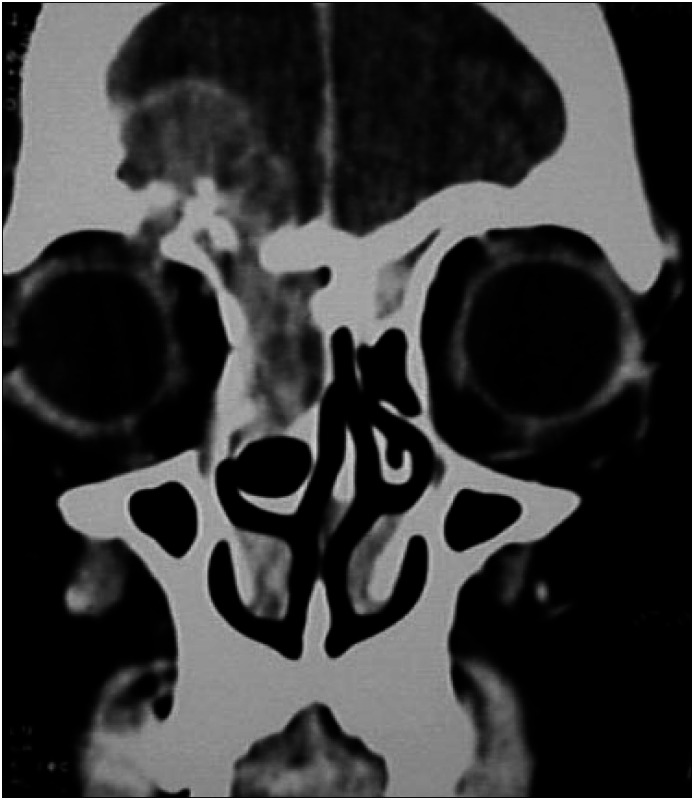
CT scan showed a soft-tissue mass involving the frontal sinus with intracranial invasion through the posterior wall. Anterior ethmoid air cells and the medial aspect of the right orbit were also involved.
